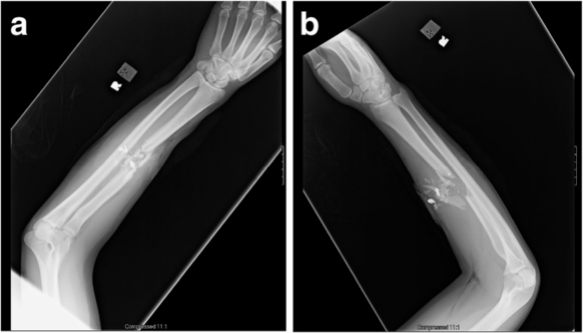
AP (a) and lateral (b) radiographs of the right forearm.
